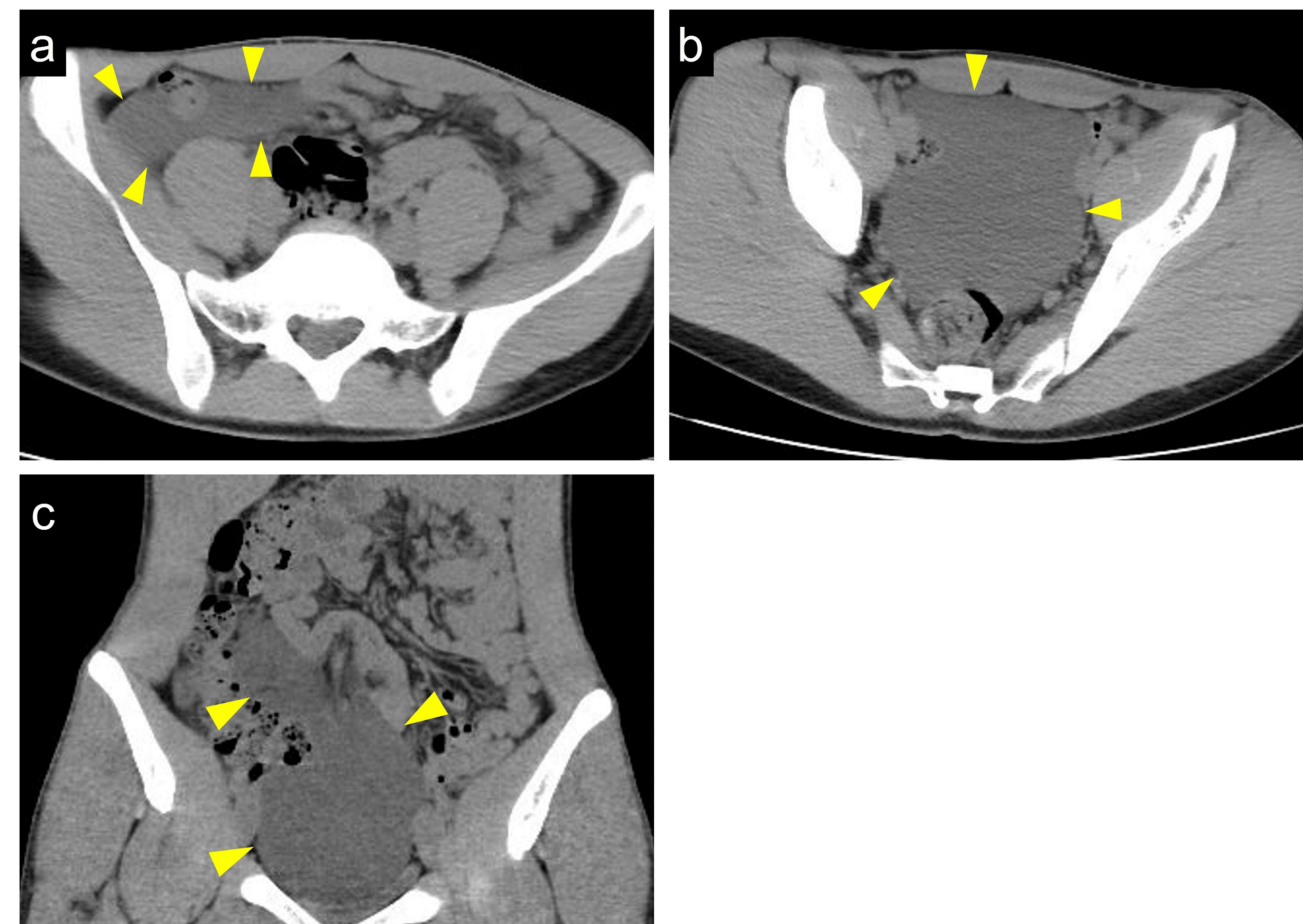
Plain computed tomography (CT) showing mesenteric lymphangioma with no remarkable change compared to two years ago (a) The axial view of CT shows the fluidlike lesion in the right iliac fossa (arrowheads). (b) Another axial view of CT shows the fluidlike lesion in pelvis (arrowheads). (c) The coronal view of CT shows the fluid-level lesion extending from the right iliac fossa to the pelvis (arrowheads)
